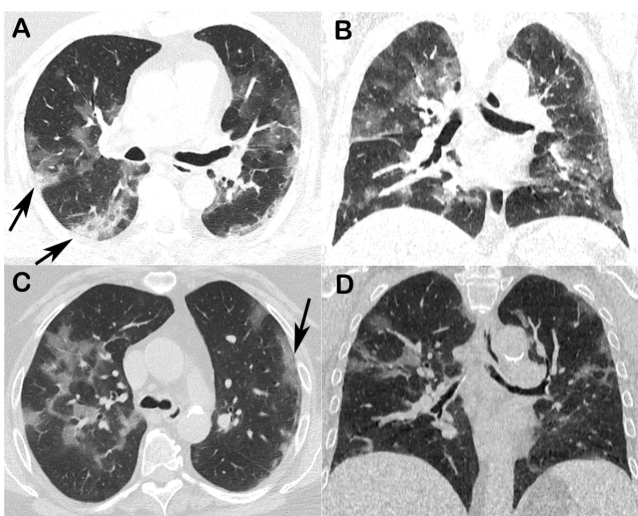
A - Patient 1, chest CT, pulmonary window, axial (A) and coronal (B) reconstructions showing multifocal areas of ground-glass opacities in both lungs, with small areas of consolidation in the right lower lobe (arrow). C and D (patient 2), chest CT with pulmonary window, showing multifocal areas of ground-glass opacities in both lungs. Note subpleural and peripheral involvement in the lingula (arrow).
